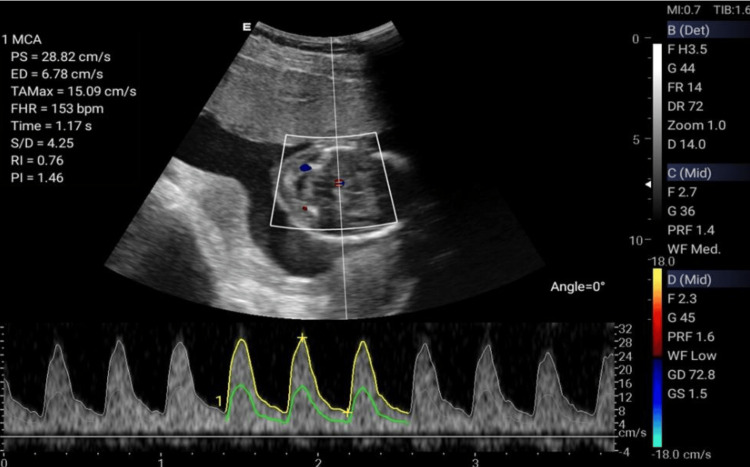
Middle cerebral artery (MCA) Doppler.
